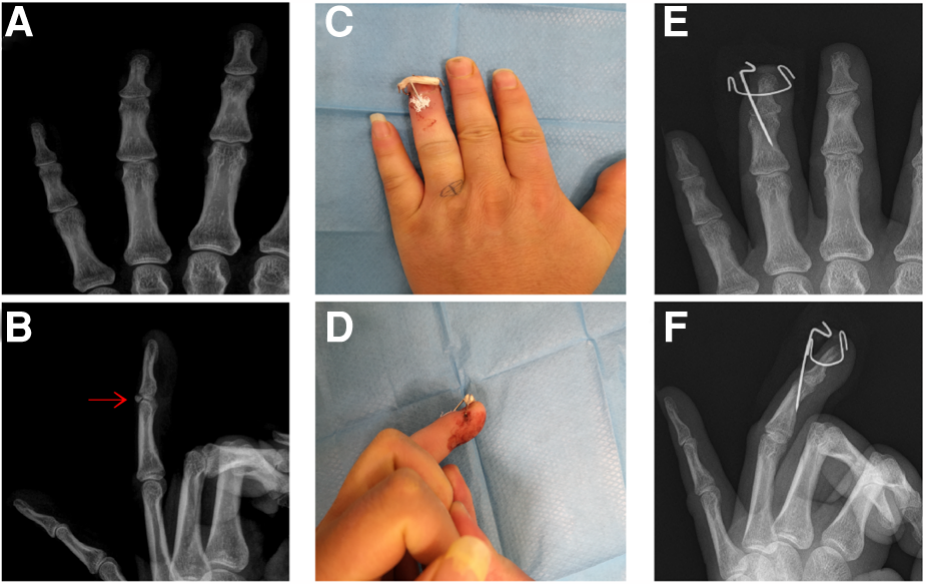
Preoperative and postoperative data of patient No.4. (A,B). Preoperative X-ray; the avulsion fracture (red arrow). (C,D). Postoperative X-ray. (E,F). Postoperative appearance.
